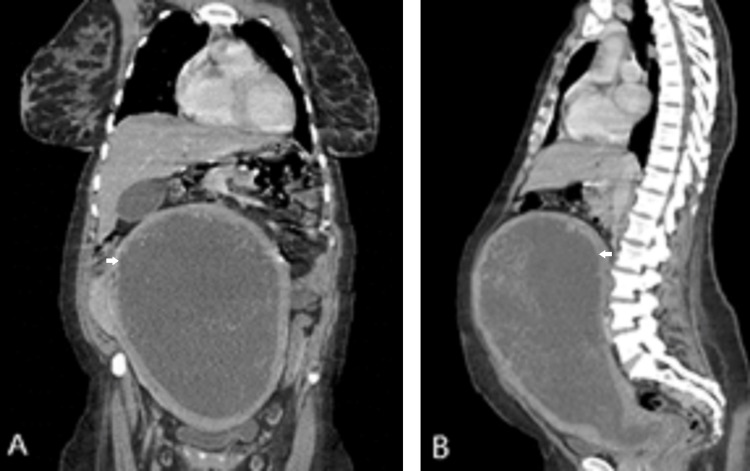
(A, B) Computed tomography scan of the abdomen and pelvis showing an enlarged uterus with heterogeneous highly vascularized content. (A) Coronal view. (B) Sagittal view.
